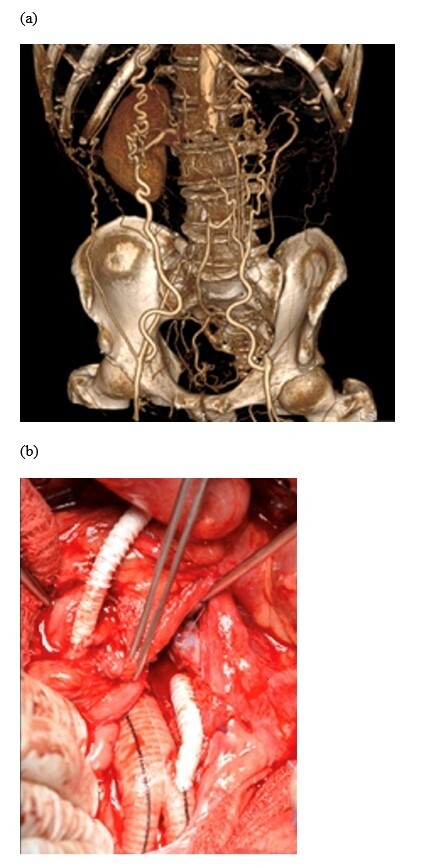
Intraoperative and imaging findings for patient #2. (a) Preoperative computed tomography angiography showing the superior mesenteric artery (SMA) and the inferior mesenteric artery occluded; (b) Intraoperative view of a polyester aortobifemoral graft with a reinforced PTFE graft from the aortic graft to the SMA.
